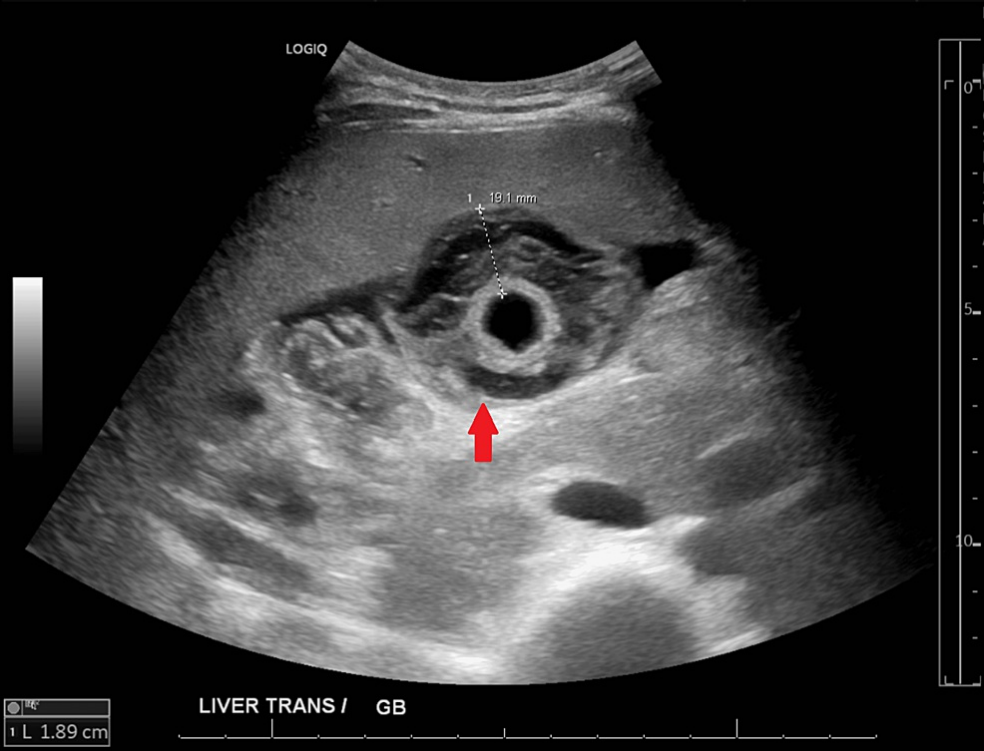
Ultrasound abdomen shows significant gallbladder mucosal thickening and edema. Gallbladder wall measures 19.1 mm in thickness (red arrow). There is no evidence of cholelithiasis.
